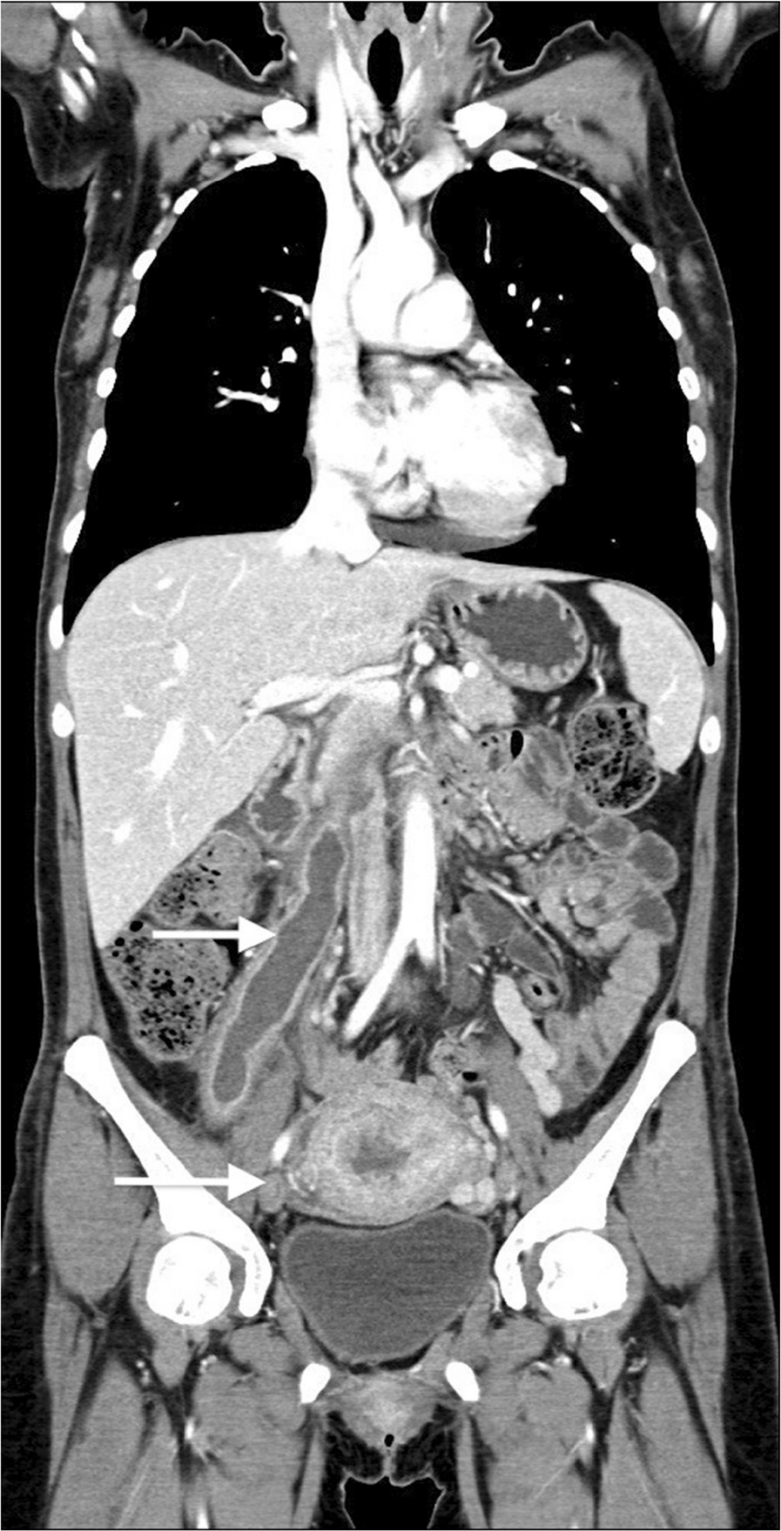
CT-scan showing complete thrombosis of IVC, right ovarian vein and pelvic varicosity.
